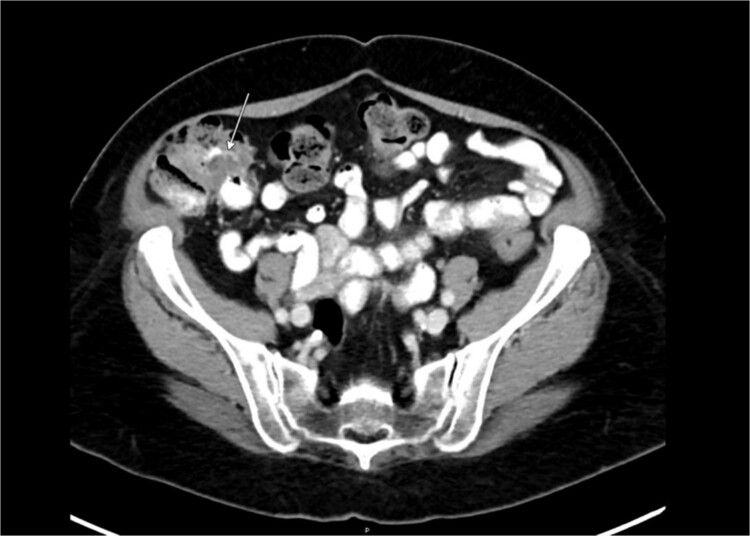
Computed tomography abdomen/pelvis—arrow indicates ascending colon mass.
